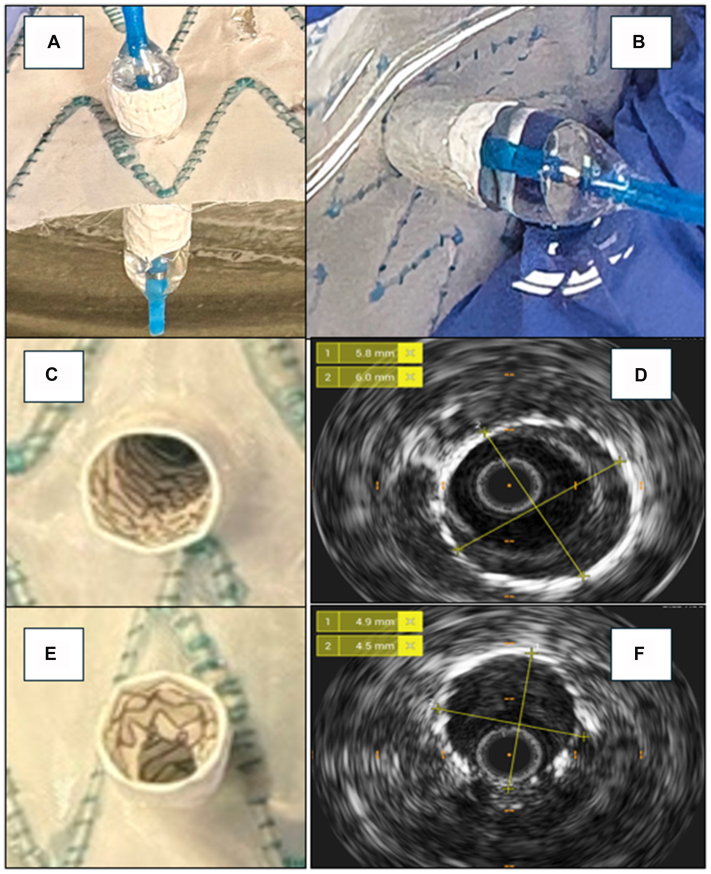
Bridging stent (BS) graft positioning. Stent graft release (A) and flaring (B). Flared BS in group A (plain balloon only) (C) and relative diameter measured with intravascular ultrasound (IVUS) at the fenestration level (D). Flared BS in group B (cutting balloon) (E) and relative diameter measured with IVUS at the fenestration level (F).
